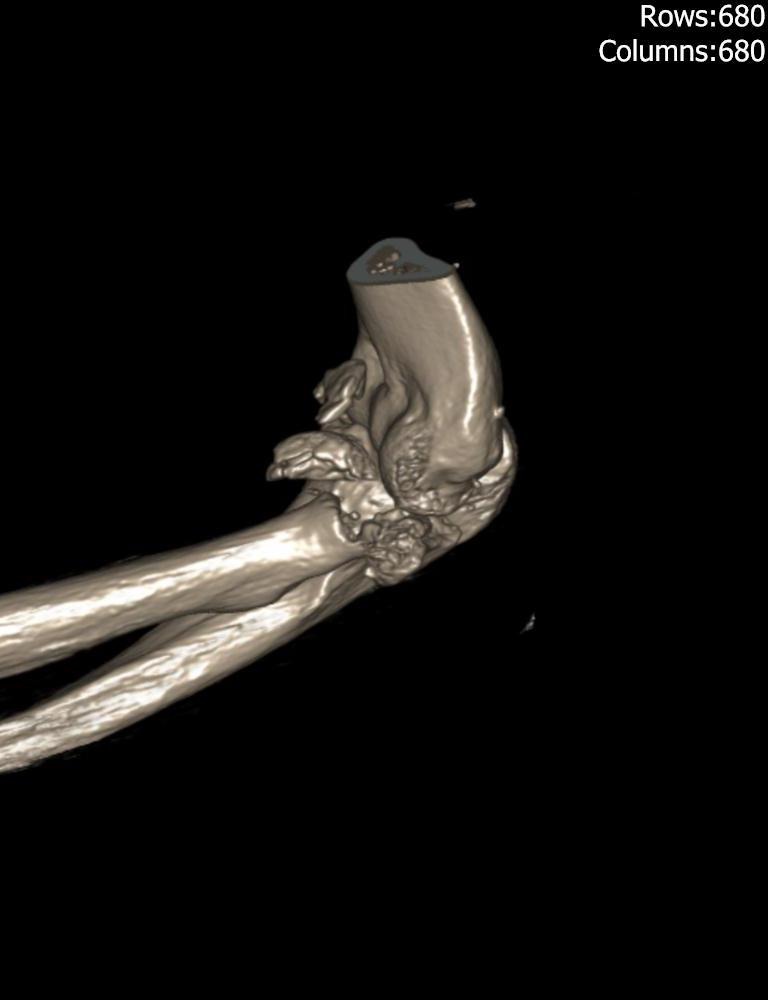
Reconstructed CT image of a type IV injury with an associated coronoid fracture.
